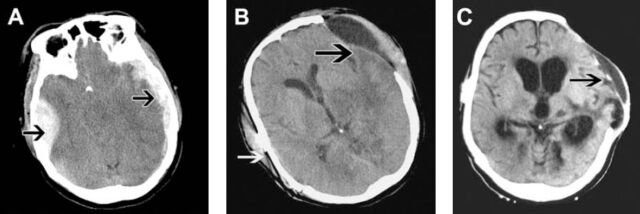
A 52-year-old male with bilateral pupil dilation underwent 2-site surgery. Non-contrast axial head CT scan shows: A) left fronto-temporal-parietal subdural hematoma and right temporal epidural hematoma; B) bilateral approach performed consecutively to remove the lesions with decompressive craniectomy performed on the left; C) follow-up, Glasgow Outcome Scale was 3.
